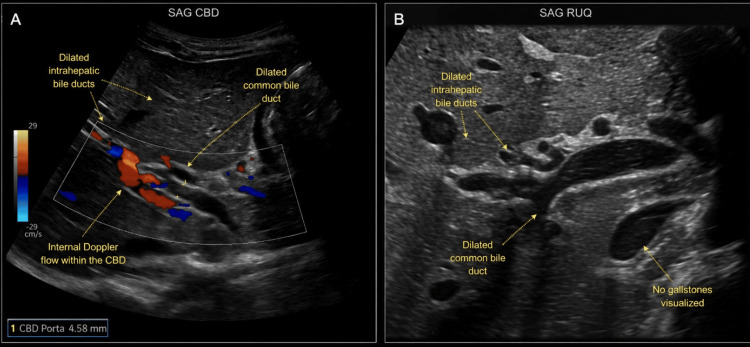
Abdominal ultrasound images demonstrating nonspecific intrahepatic and extrahepatic biliary ductal dilatation (A) Sagittal color Doppler view of the common bile duct showing mild common bile duct prominence with adjacent intrahepatic biliary ductal dilatation. (B) Sagittal grayscale right upper quadrant view demonstrating intrahepatic biliary ductal dilatation and extrahepatic duct prominence without evidence of cholelithiasis or choledocholithiasis. Trace ascites was present (not shown). Visualization of the pancreas was limited by overlying bowel gas.
